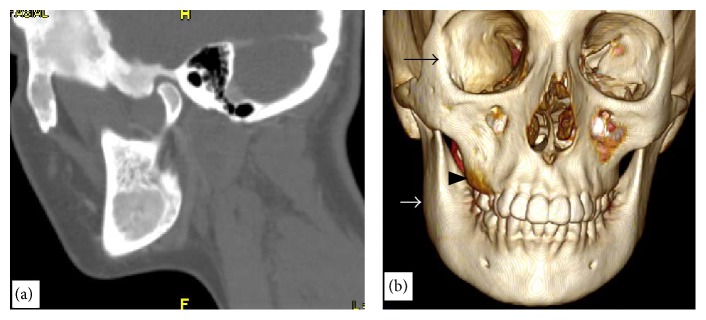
(a) Sagittal reformatted CT images showing expansive mixed lesions involving the mandible, temporal bone, and zygomatic bone. (b) 3D CT images showing the CFD affecting the right side of the mandible (white arrow), in the right maxilla (black arrow head), in the right lateral wall of the orbit (black arrow).
